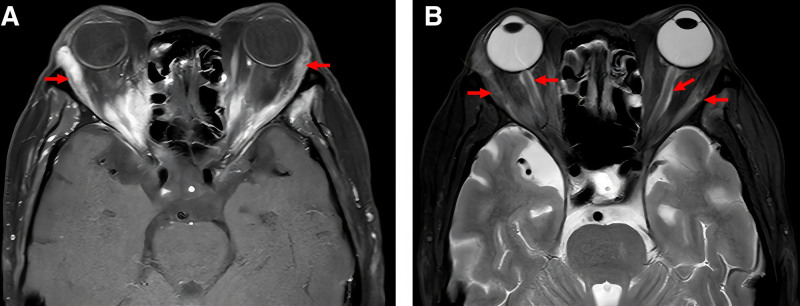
Plain + contrast-enhanced orbital MRI. (A) Bilateral lacrimal gland enlargement with obvious uniform enhancement. (B) Slight bilateral extraocular muscle enlargement with obvious margin enhancement, linear enhancement observed in the bilateral optic nerve sheath regions.
